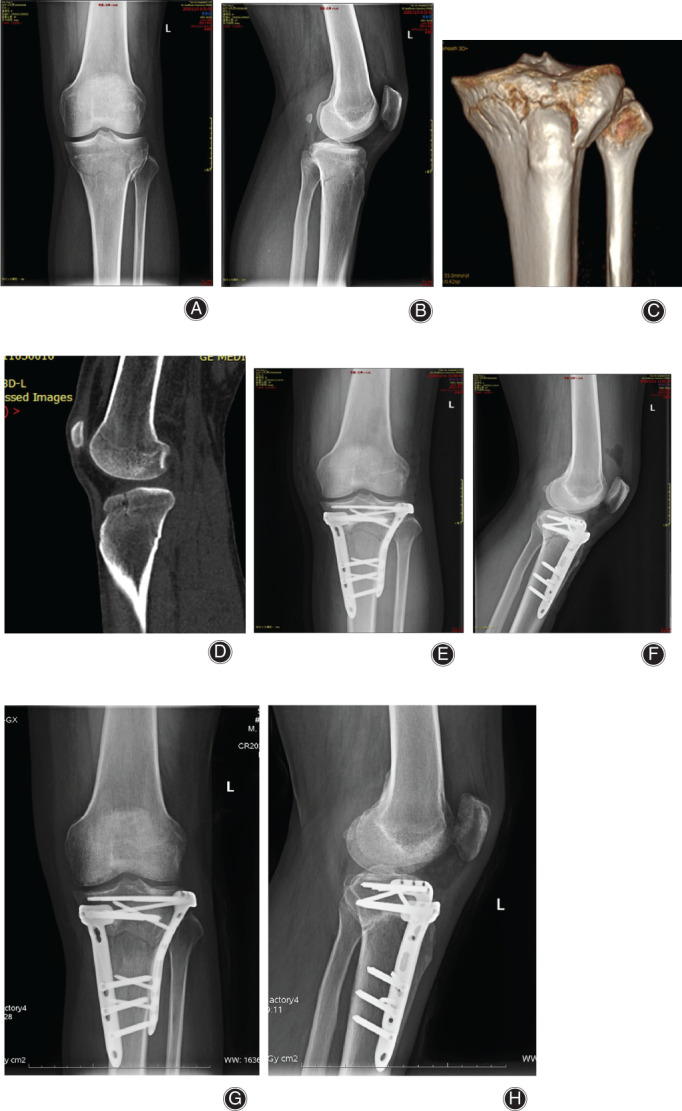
Anteromedial + anterolateral tibial plateau fracture. Male, 33 years old. (A, B) X‐ray shows medial and lateral tibial plateau fracture, and the loss of posterior slope of tibial plateau. (C, D) 3D CT shows the compression of anteromedial and anterolateral tibial plateau, and posterior tension fracture. (E, F) The fracture was reduced and fixed with an L‐shaped plate in the lateral tibia and a Tomofix plate in the anteromedial tibia. (G, H) The fracture healed and satisfactory reduction was achieved 6 months postoperatively.
